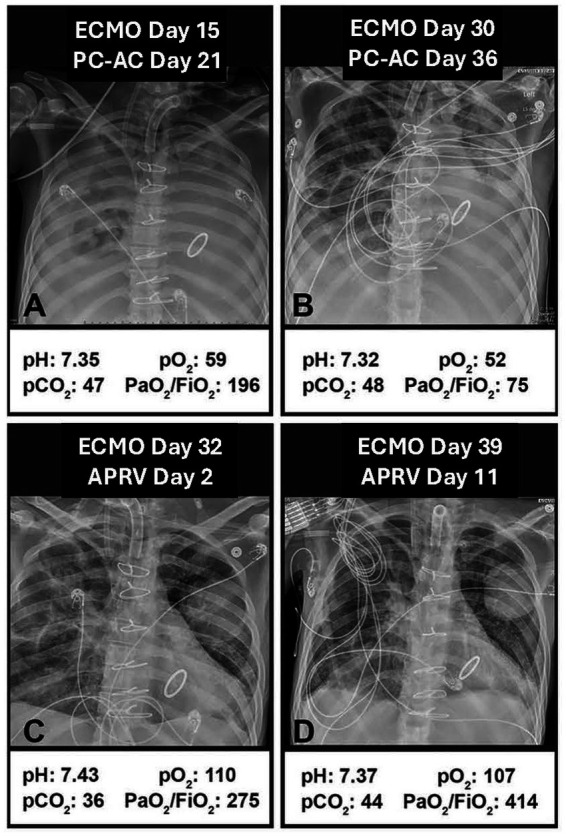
A 35-year-old man with catastrophic antiphospholipid syndrome and respiratory failure. (A) ARDSnet low tidal volume ventilation with pressure–control assist–control (PC-AC) mode, day 21, with extracorporeal membrane oxygenation (ECMO), day 15, and (B) day 36 on PC-AC with ECMO,. Transitioned to APRV on day 36. (C) 2 days on APRV, the lungs opened (clearer chest X-rays), and arterial blood gases (ABGs) improved. (D) After 11 days on APRV, the chest X-ray was clear, the ABGs normalized, and the patient was removed from ECMO and mechanical ventilation (54). This case demonstrates the gradual ‘ratcheting’ open of the lung tissue over several days with the use of the APRV (49).
